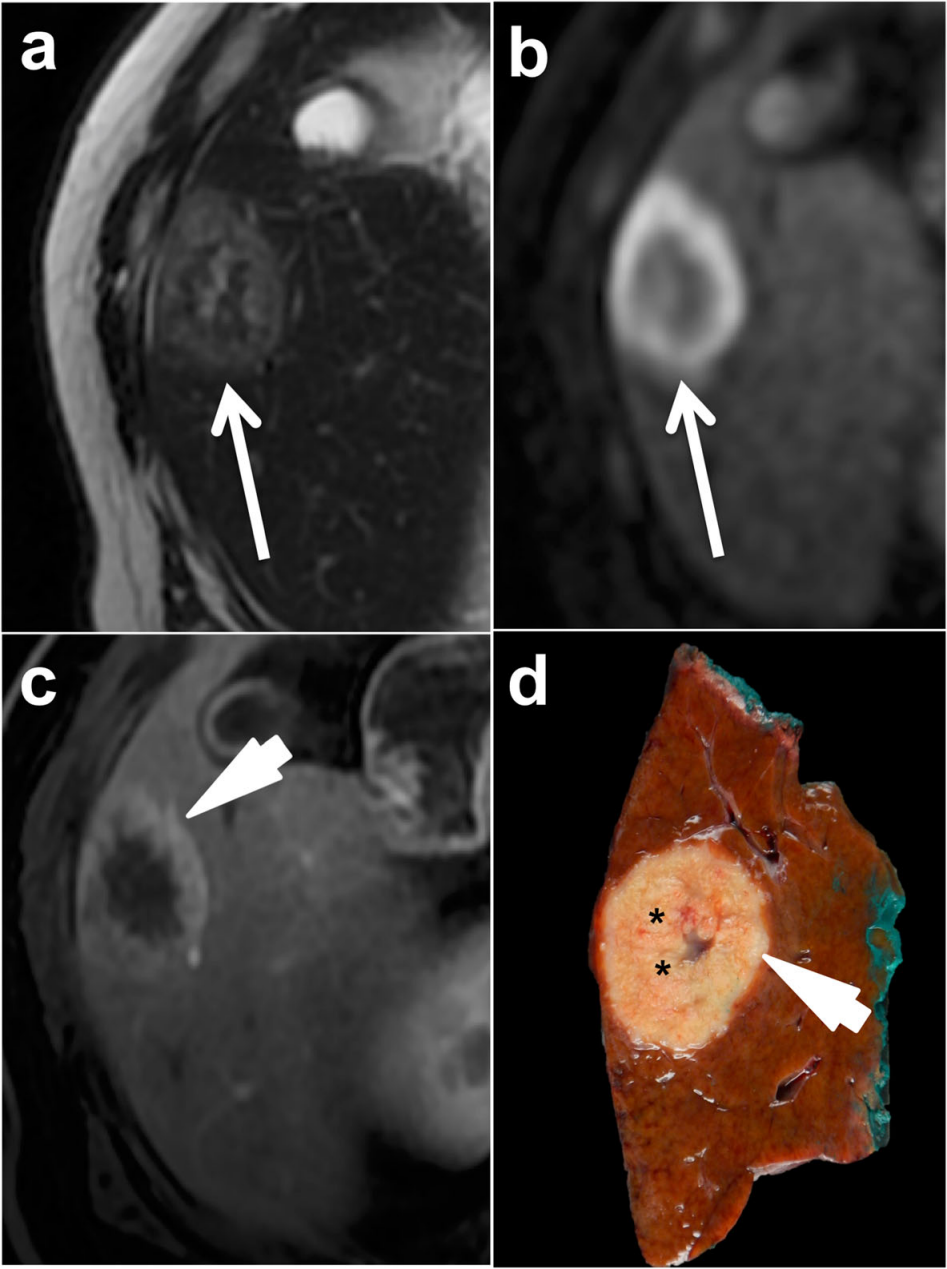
Typical MR appearance of non-otherwise specific (NOS) adenocarcinoma liver metastases in a 55-year-old male patient with colon cancer. Fast spin echo T2-weighted image (a) shows a 50 mm lesion (arrow) in the right liver lobe with a mild hyperintense rim and a central heterogeneous area. On diffusion-weighted imaging (b 600 s/mm^2^) (b) the lesion has a peripheral hyperintense rim (arrow). The lesion also shows peripheral enhancement (arrowhead) on extracellular gadolinium chelate-enhanced fat-saturated gradient recall echo T1-weighted sequences obtained during the hepatic arterial phase (c). Gross pathology (d) confirmed the central necrosis of the lesion (black stars) and the predominantly peripheral tumor proliferation (arrowhead)
